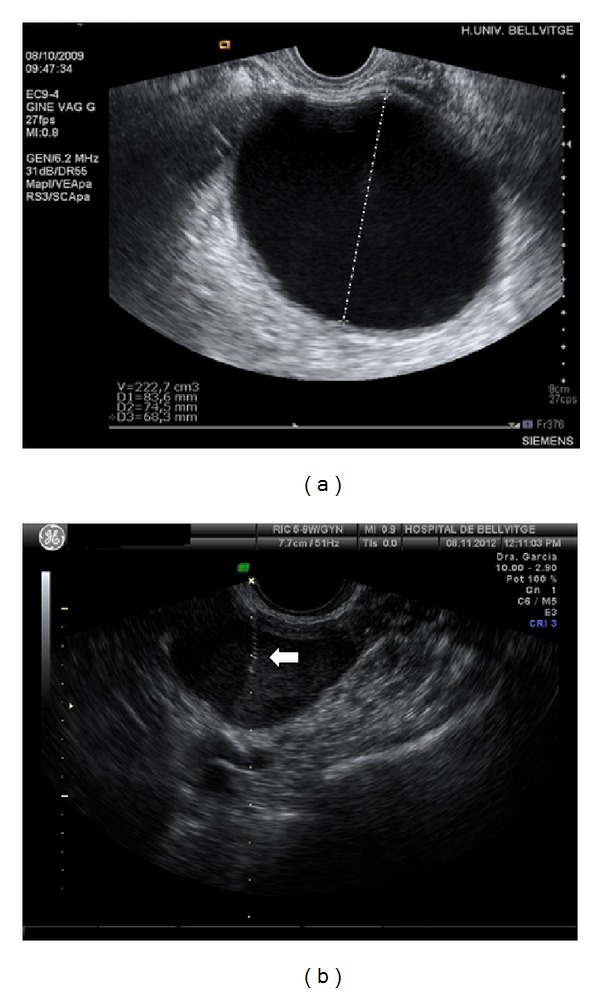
Transvaginal ultrasound-guided aspiration of an endometrioma. (a) Endometrioma measured before aspiration. Dotted line indicates one of the diameter measurements. (b) Endometrioma being aspirated. The arrow indicates the end of the needle inside the cyst. Note the reduction in the volume after aspiration.
